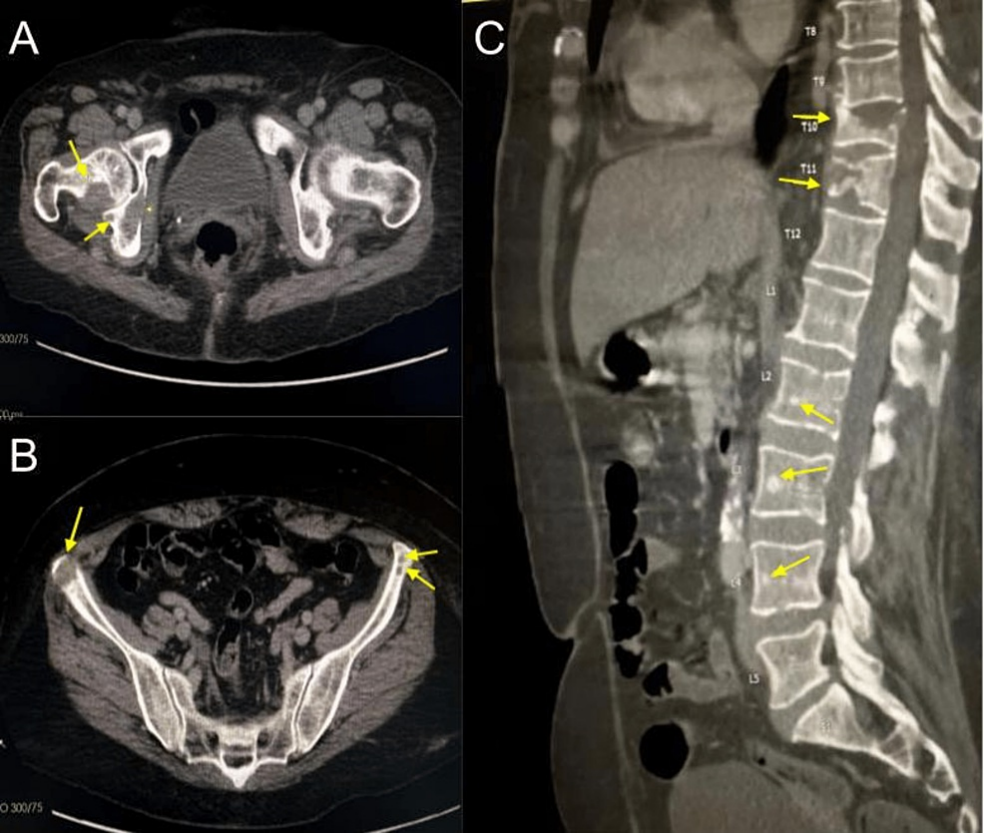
(A) CECT of the pelvis (axial view): destructive lytic lesions in the right femoral head and posterior column of the right acetabulum with an underlying pathological fracture (not shown). (B) CECT of the pelvis (axial view): bilateral lytic lesions involving the anterior iliac crests, expansile on the left. (C) CECT of the pelvis (sagittal view): diffuse bony metastases with multiple lytic and blastic lesions throughout the thoracolumbar spine. Pathological fracture seen at T10 with mild vertebral height loss. CECT: contrast-enhanced computed tomography
